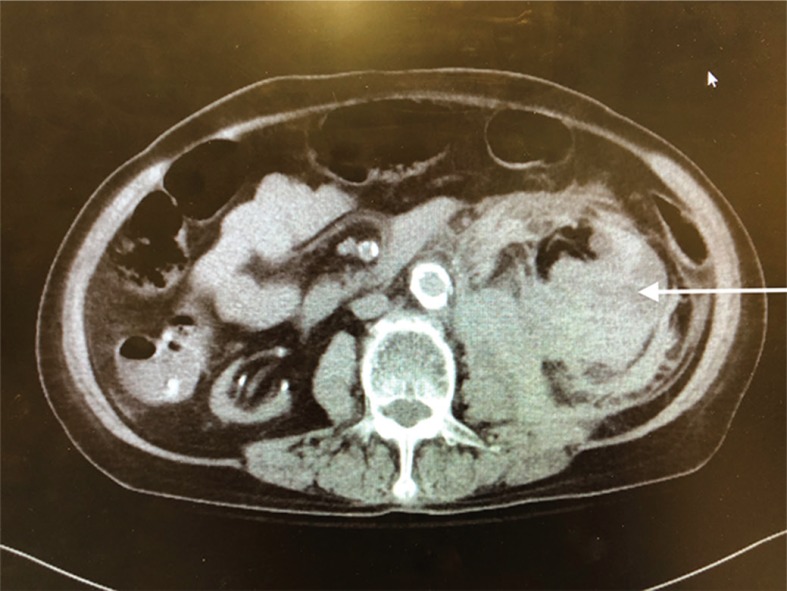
Axial CT image of left retroperitoneal hemorrhage.The arrow refers to the large retroperitoneal hematoma.
